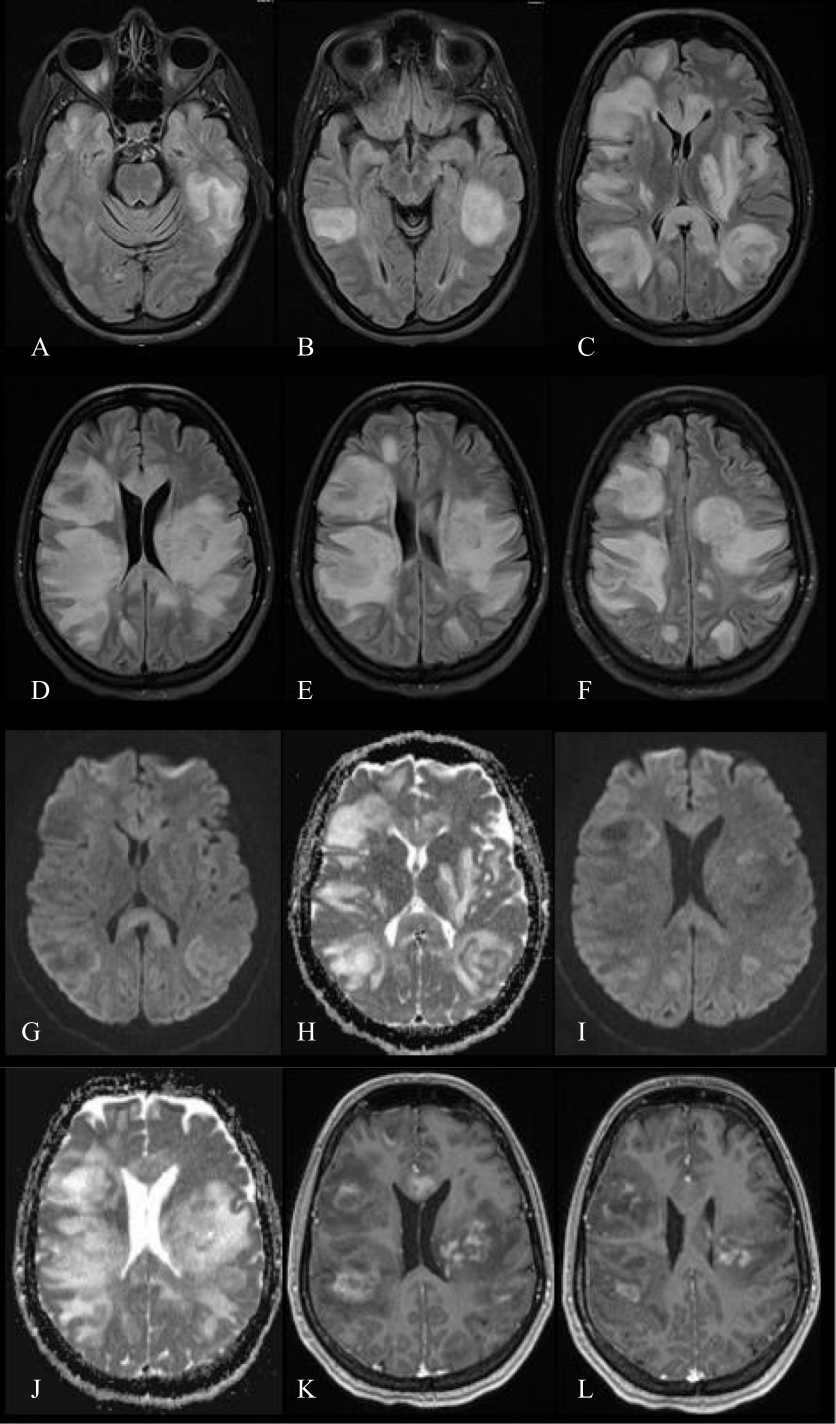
Follow-up MRI of the patient (2020.11.06) with further expansion of the lesions, broad edematous areas and ventricular compression. A-F axial T2W FLAIR images; G, I axial DW; H, J axial ADC; K, L axial T1W with contrast
